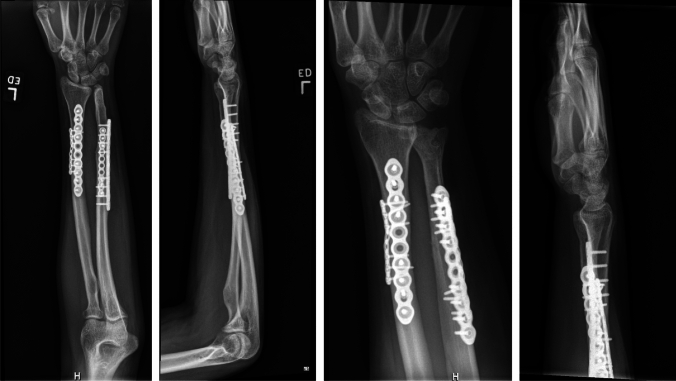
Postoperative radiographs at 16 months demonstrating maintenance of reduction and osseous union
